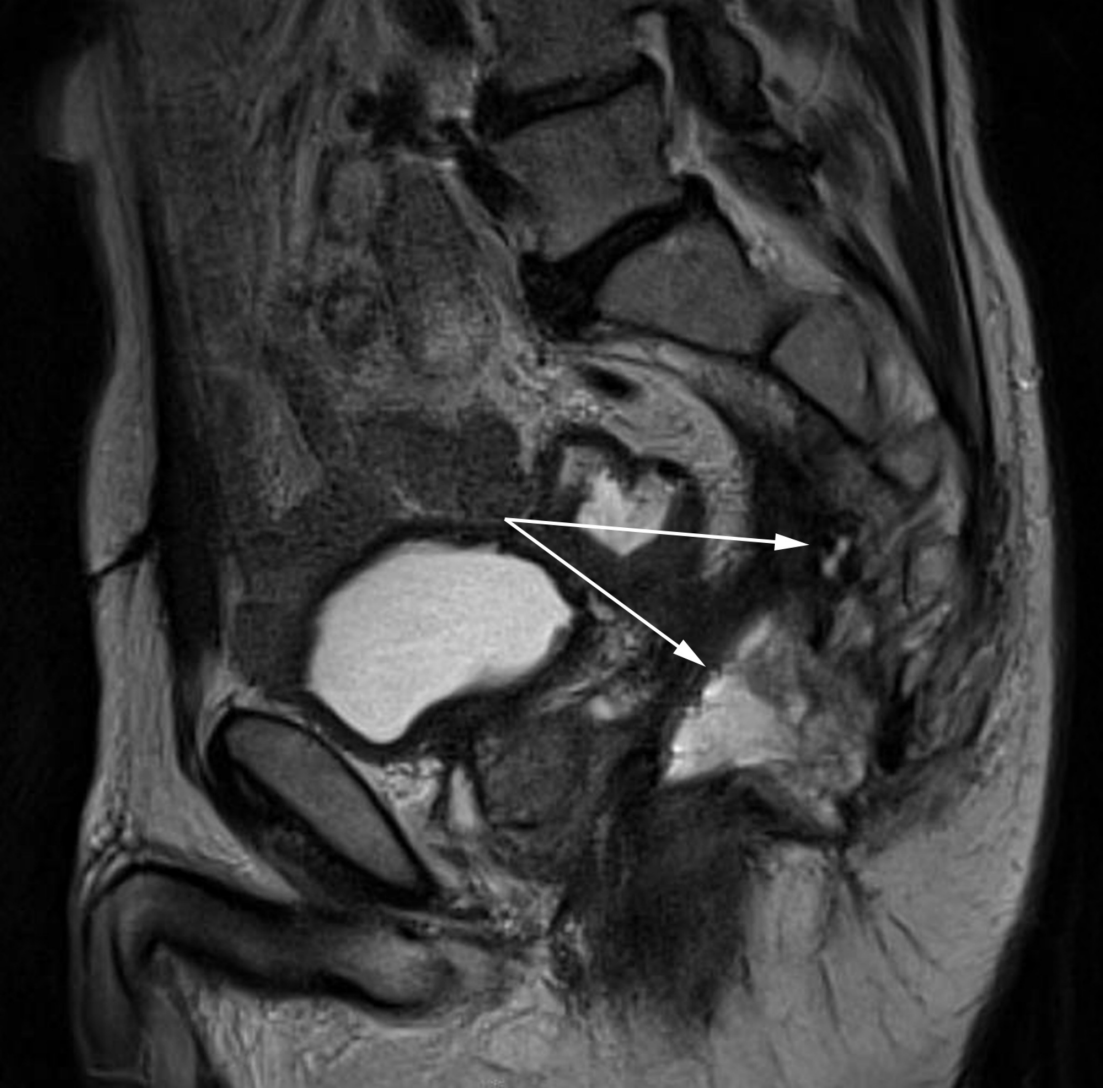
T2 MRI showing posterior wall rectal defect with a presacral abscess
